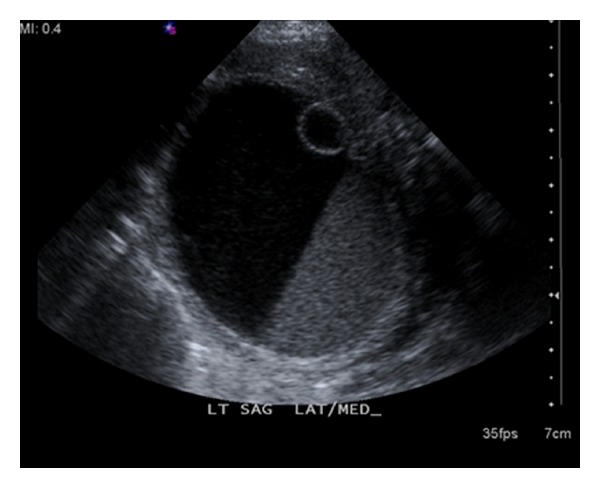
A sagittal gray scale image of the left ovary shows a 4.5 × 5.6 × 4.5 cm cystic mass with low-level-dependent echoes, consistent with internal hemorrhage. A smaller cyst is seen internally, measuring 1.0 cm in diameter. The findings were identical when compared to the prenatal ultrasound. Similar findings were seen on the parental ultrasound.
